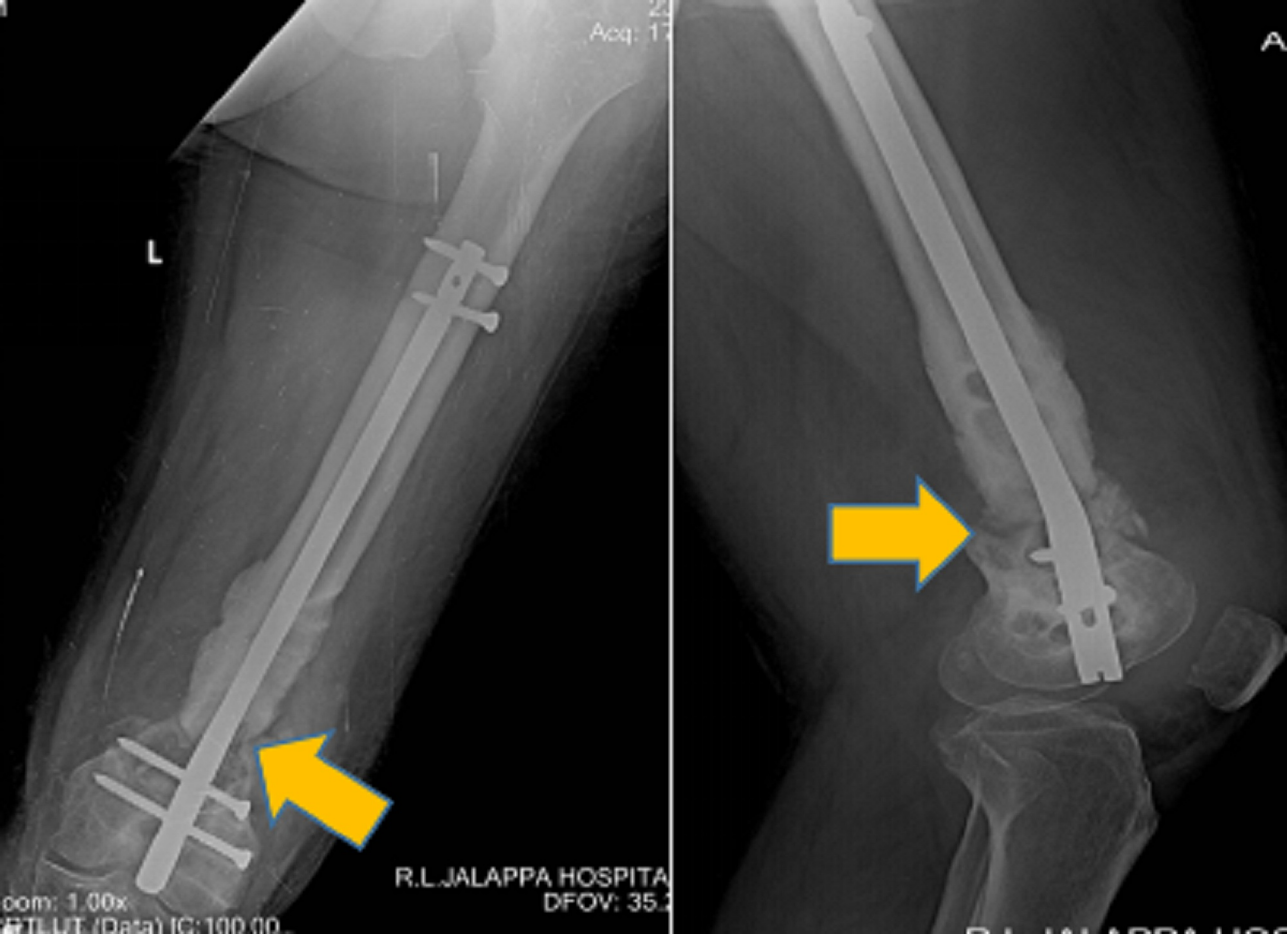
Six-month post-operative, plain antero-posterior left thigh radiograph and lateral view showing fracture uniting with distal femoral nail in place
